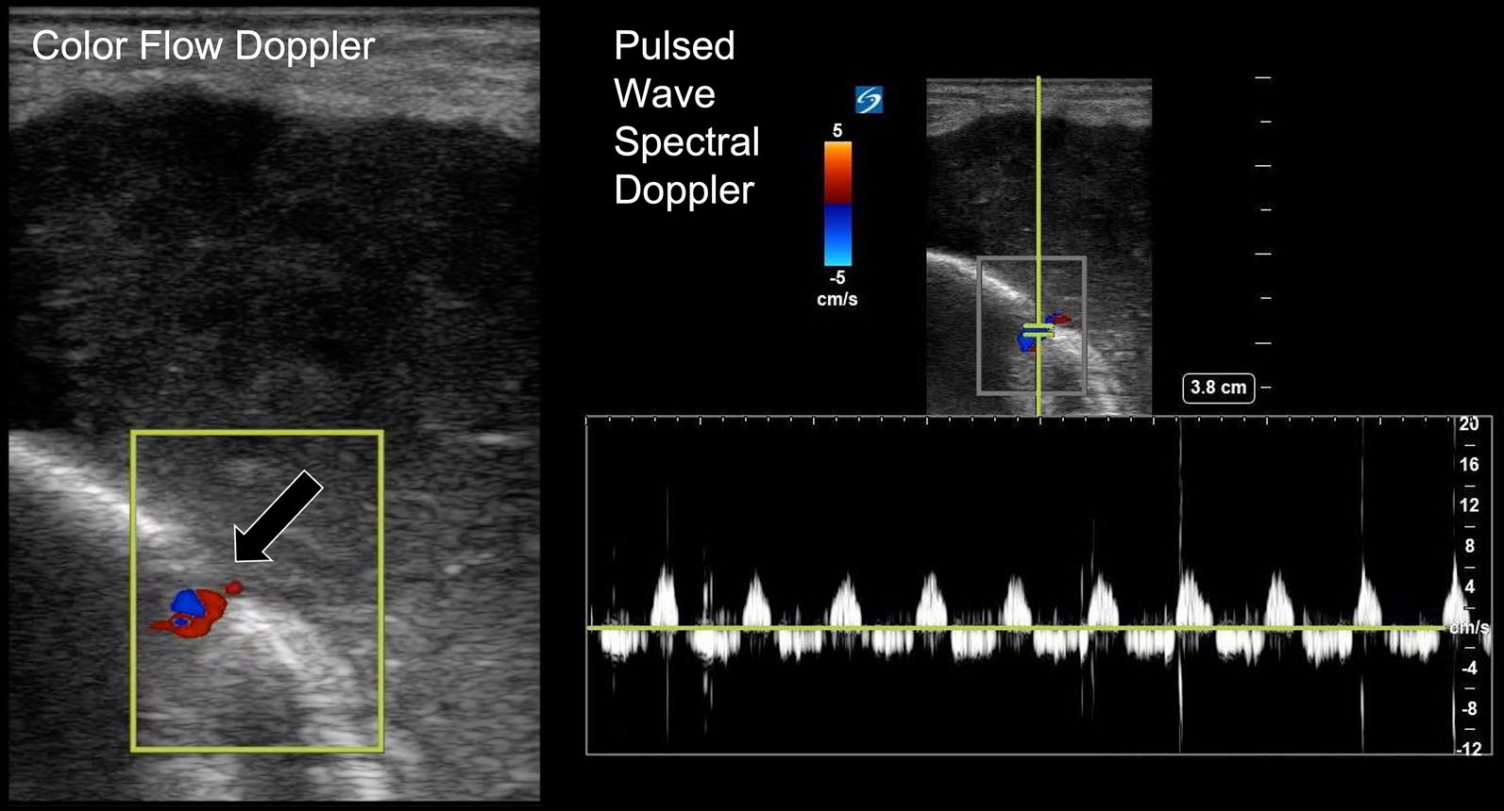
Point-of-care ultrasound demonstrating alternating color flow and pulsed wave spectral Doppler through the cortical defect (black arrow)
